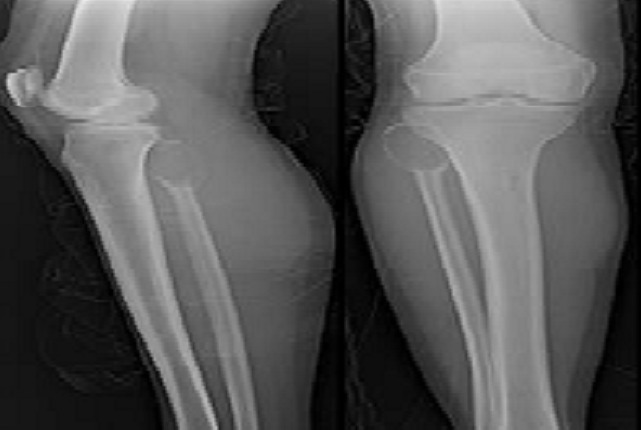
Radiograph showing an osteolytic lesion of the head of the fibula associated with intralesional few thin walls
